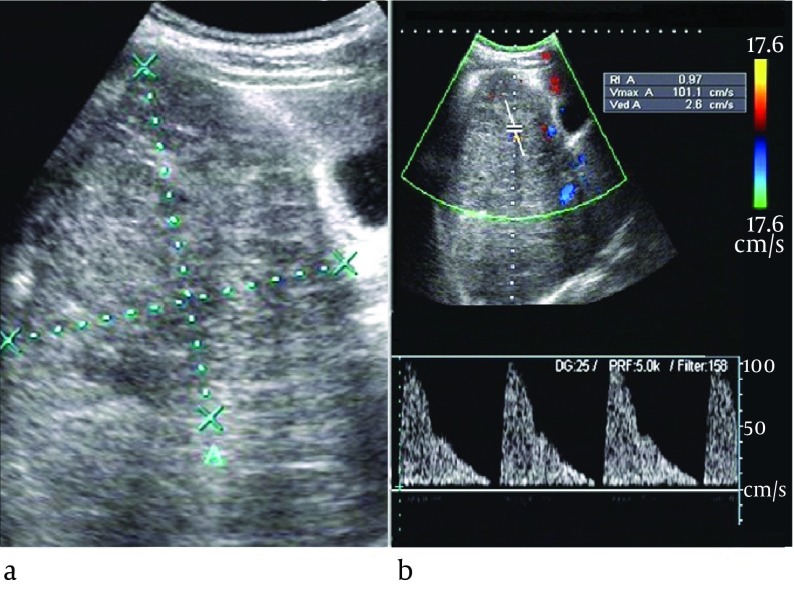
Hepatoblastoma in a 1-Year-Old Girl (a) Gray-scale US image showed an isoechoic nodule (calipers) 5.8 cm in size, in segment 5 of the liver. (b) Spectral Doppler showed arterial flow with PSV of 101.1 cm/s and RI of 0.97
